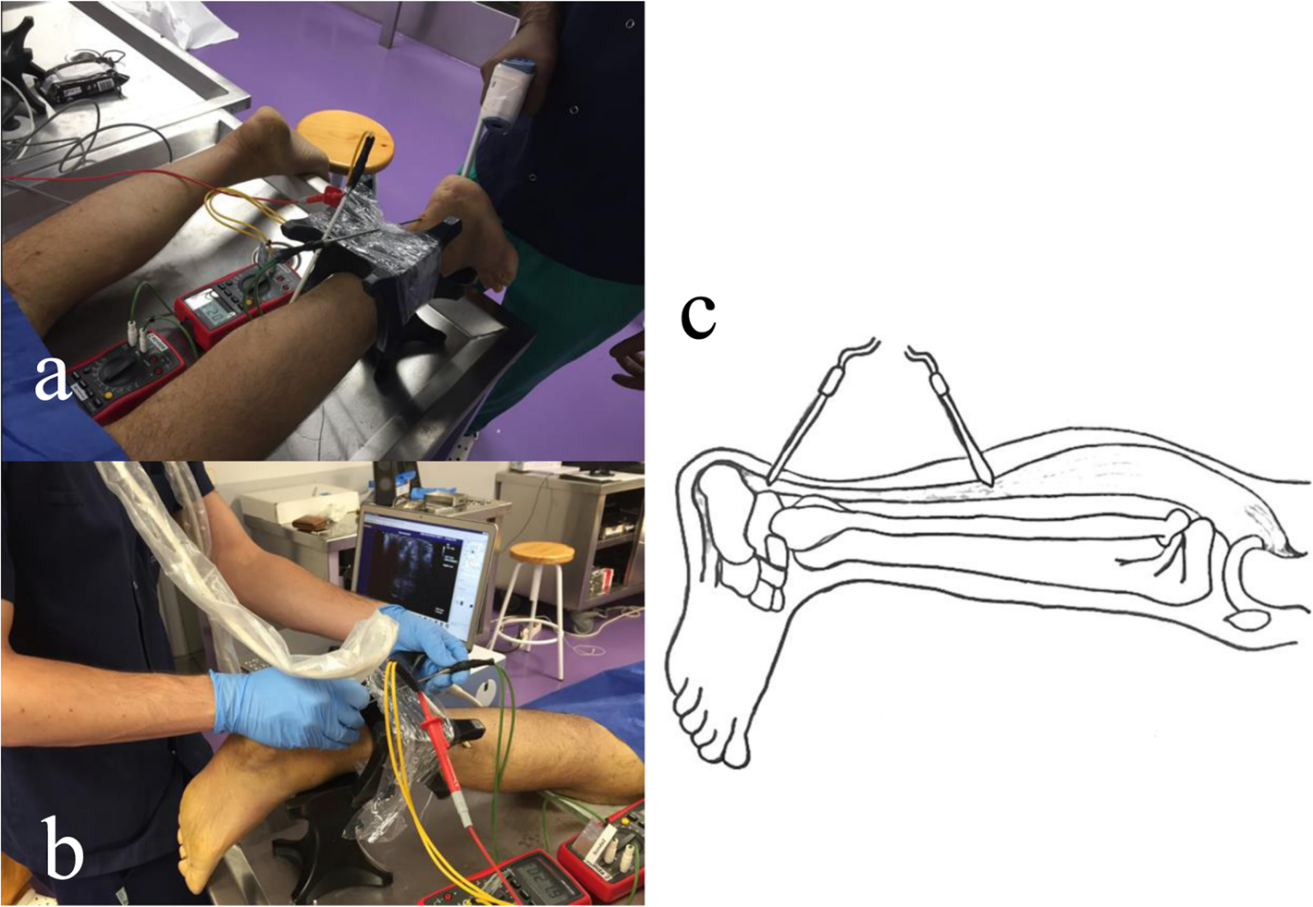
a Temperature measurement with digital thermometer; b Thermocouple placement under ultrasound guidance; c Thermocouple position
